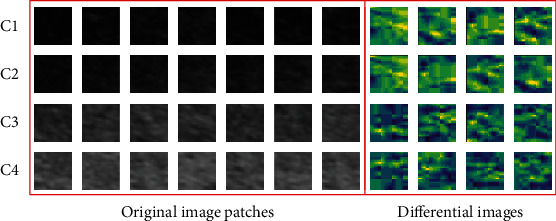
Input windows with  *w* = 28, from original ultrasonic images and differential images. The *C*1, *C*2, *C*3, and *C*4 correspond to the normal liver, low-grade fatty liver, moderate grade fatty liver, and severe fatty liver.
